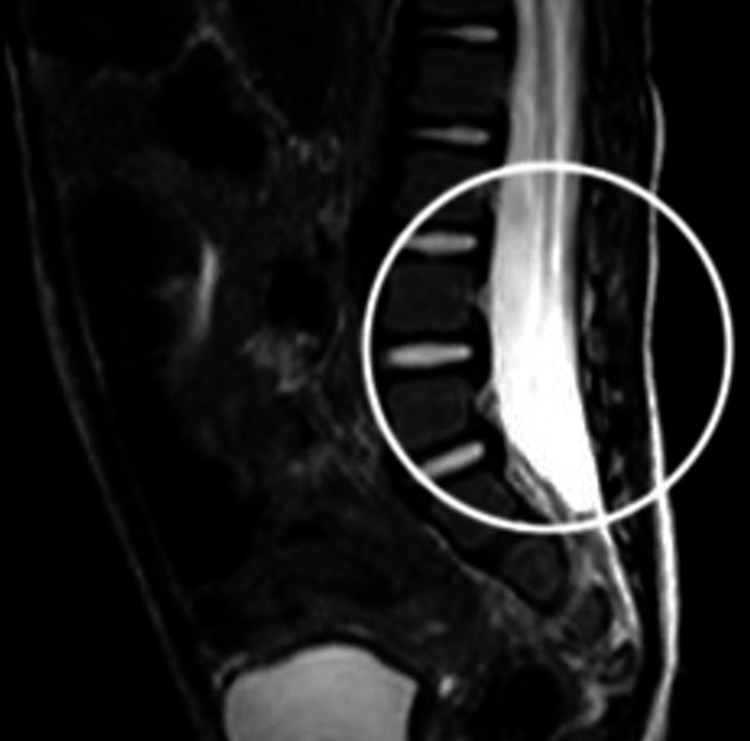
T2 weighted sagittal view of magnetic resonance imaging of the lower spine showing incidental finding of dural ectasia (white circle) in a child with Cowden syndrome.
